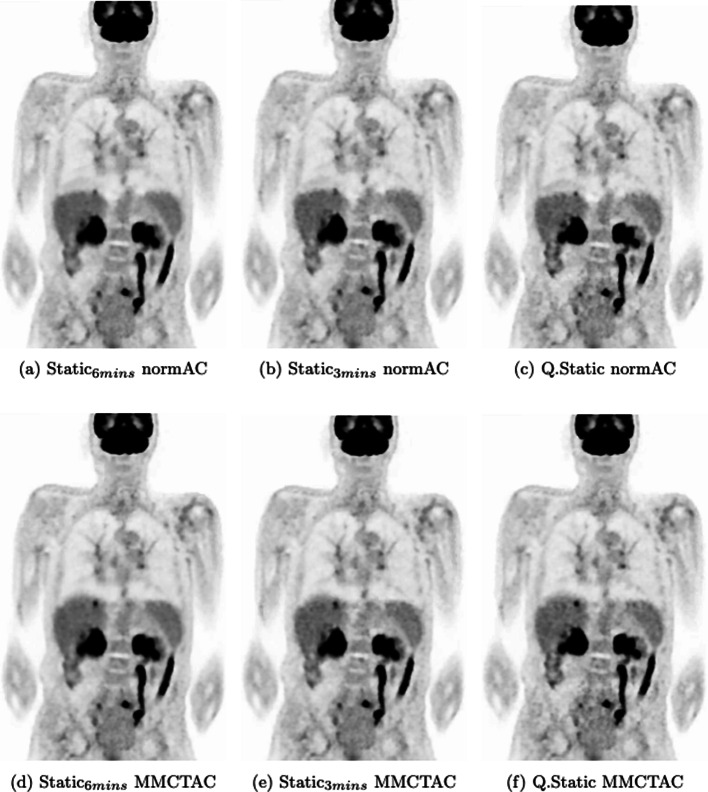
Comparison of PET/CT reconstructions using normAC a–c and MMCTAC d–f, taken from the same coronal image slice. The effect of the MMCTAC improving the localization of the lesion within the liver is clearly visible. Static 6 min reconstructions exhibited normAC lesion SUVmax = 3.2, MMCTAC lesion SUVmax = 5.3 (on SUV scale 0-8). These images are reconstructed from a patient included in this study, scanned at Oxford University Hospitals
